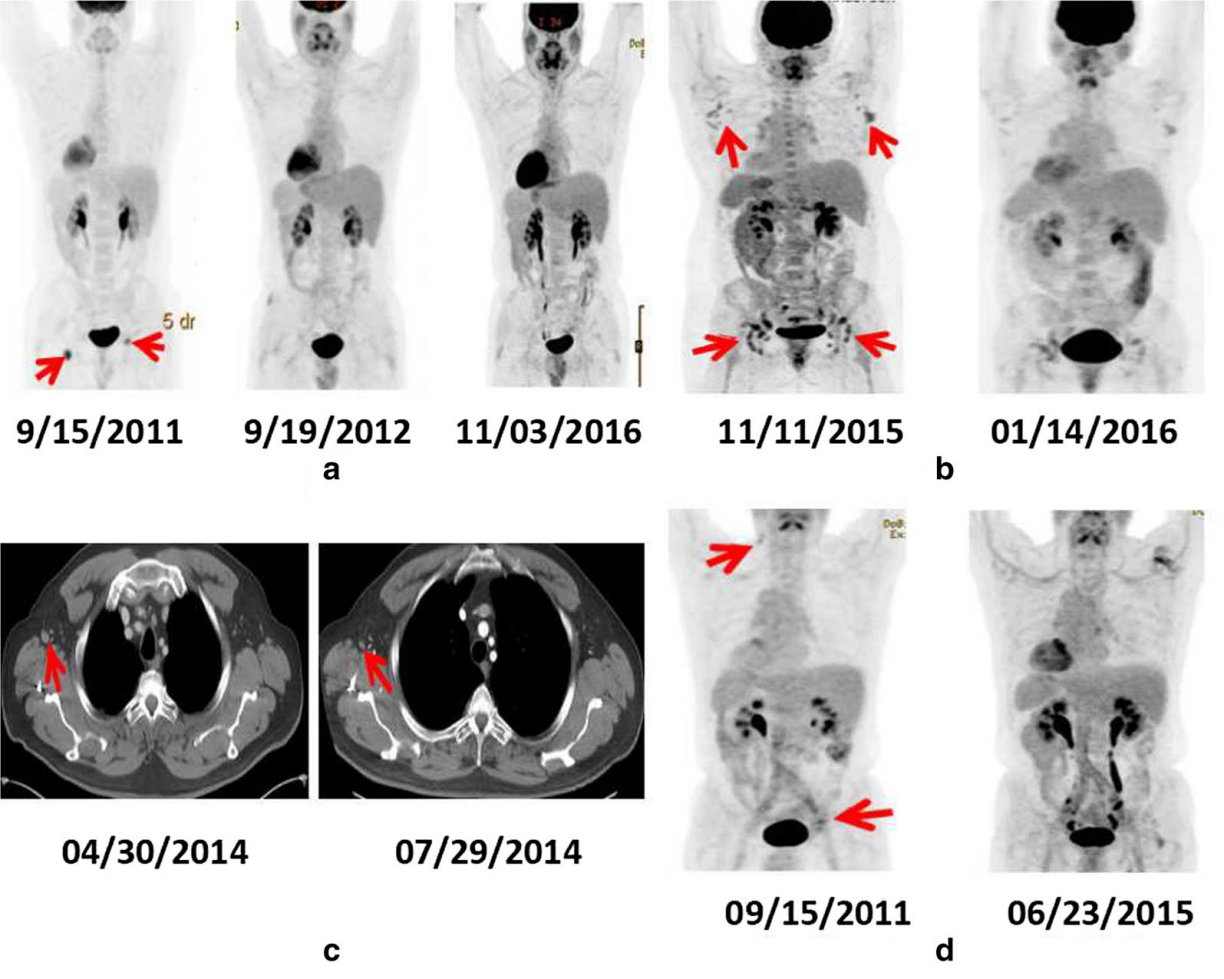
Spontaneous regression of four cases of mantle cell lymphoma. a Comparison of positron emission tomography combined with computed tomography (PET/CT) images on September 15, 2011, September 19, 2012, and November 3, 2016, showing the resolution of fluorodeoxyglucose (FDG)-avid lymph nodes in a 48-year-old man with MCL (case 1). b Comparison of PET/CT on November 11, 2015, and January 14, 2016, showing the resolution of FDG uptake in the right axillary, prevascular, left obturator, and bilateral inguinal lymph nodes in a 44-year-old woman with MCL (case 2). c Chest CT with contrast on April 2014 showed right axillary lymph node enlargement (1.3 × 1.1 cm), whereas chest CT on July 2014 showed a decrease in size (0.8 × 1.0 cm) in a 63-year-old man with MCL (case 3). d PET/CT on September 2011 showed low-grade FDG-avid inguinal and left posterior cervical triangle adenopathy (Standardized uptake value was 2.6), whereas PET/CT on June 2015 showed resolution in a 68-year-old man with MCL (case 4). Red arrows indicate FDG-avid lymph nodes
